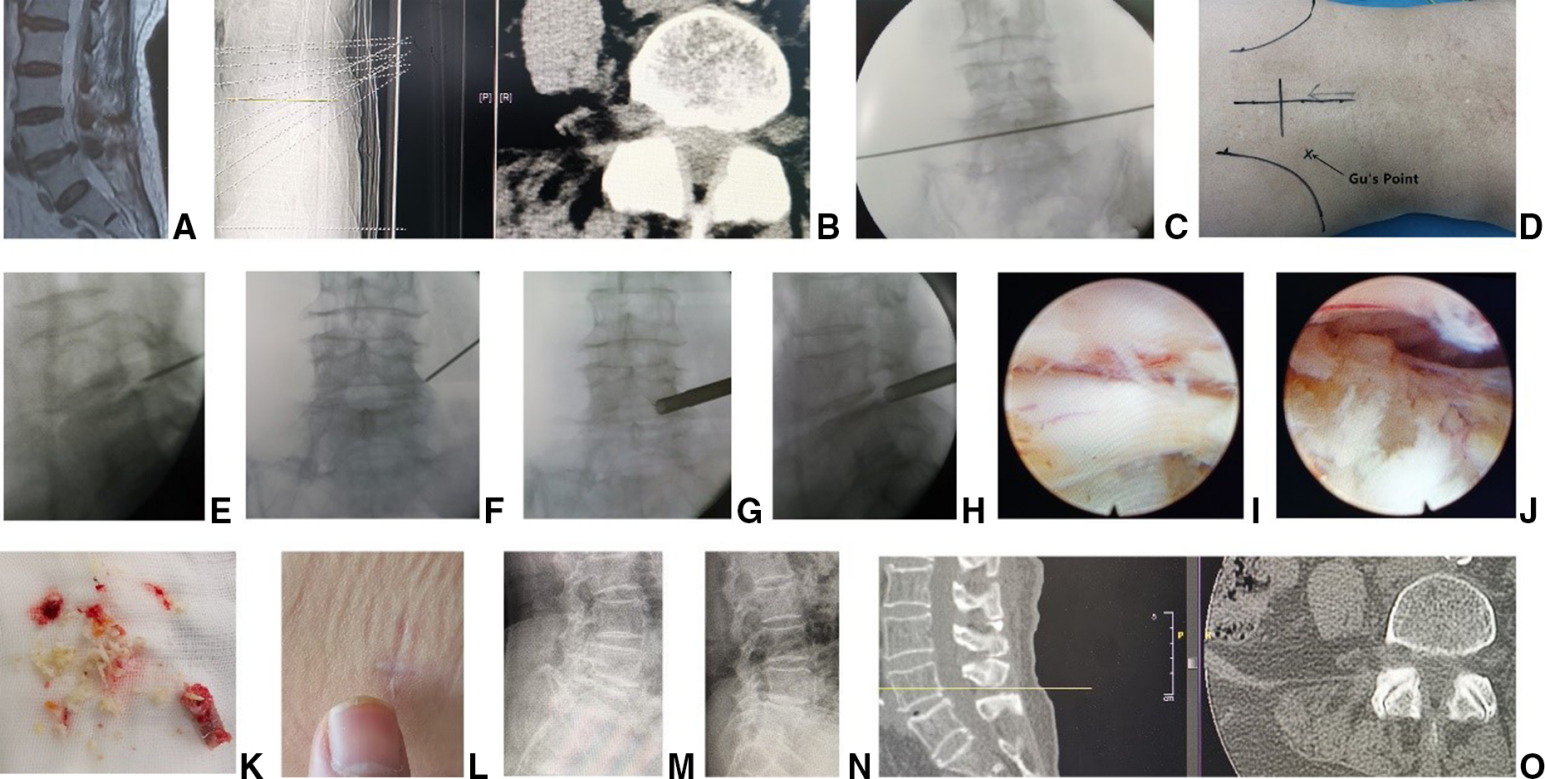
Female patient of 89 seats with LDD aL4 5 had bilateral asymmetric leg pain and the right side is more seven preoperative (A) sagittal MR and (B) CT images showed L4/5 disc herniation, lateral recess stenosis and neurologic compression. The procedure of PIES was undertaken for the patient. A transverse line bisecting the disc was draw along the metal rod which was placed transversely across the center of the target disc on (C) posteoanterior C-arm view. (D) Photography showed the surface marking of anatomic disc center identified by the intersection of transve: line and longitudinal midline, which was the aiming reference point of puncture, and the entrance point of puncture (Gu's point) located at the corner of flat back turning to lateral side. During puncture, once resistance disappeared, the C-arm view was taken to ensure that the tip of puncture needle was in the intracanal area clot to the posterior wall of disc on (E) lateral x-ray and near the lateral border of pedicle on (F) posteoanterior x-ray. During press down enlargement of foramen, when resistance disappeared, the tip of reamer should exceed the medial border of pedicle on (G) posteroanterior C-arm view and reach clot to the posterior wall of target disc on (H) lateral C-arm view. Under (I,J) endoscopic view, the bilateral compressed nerve roots were freed after (K) the hypertrophic ligamentum flavum and herniated disc were removed. (L) The picture showed the mini incision for PIES 2 years after surgery. No new lumbar instability was found on postoperative (M) hyperextension and (N) hyperflexion lateral x-ray image. (O) CT images showed the resected articular process and enlargement of L4/5 right foramen.
